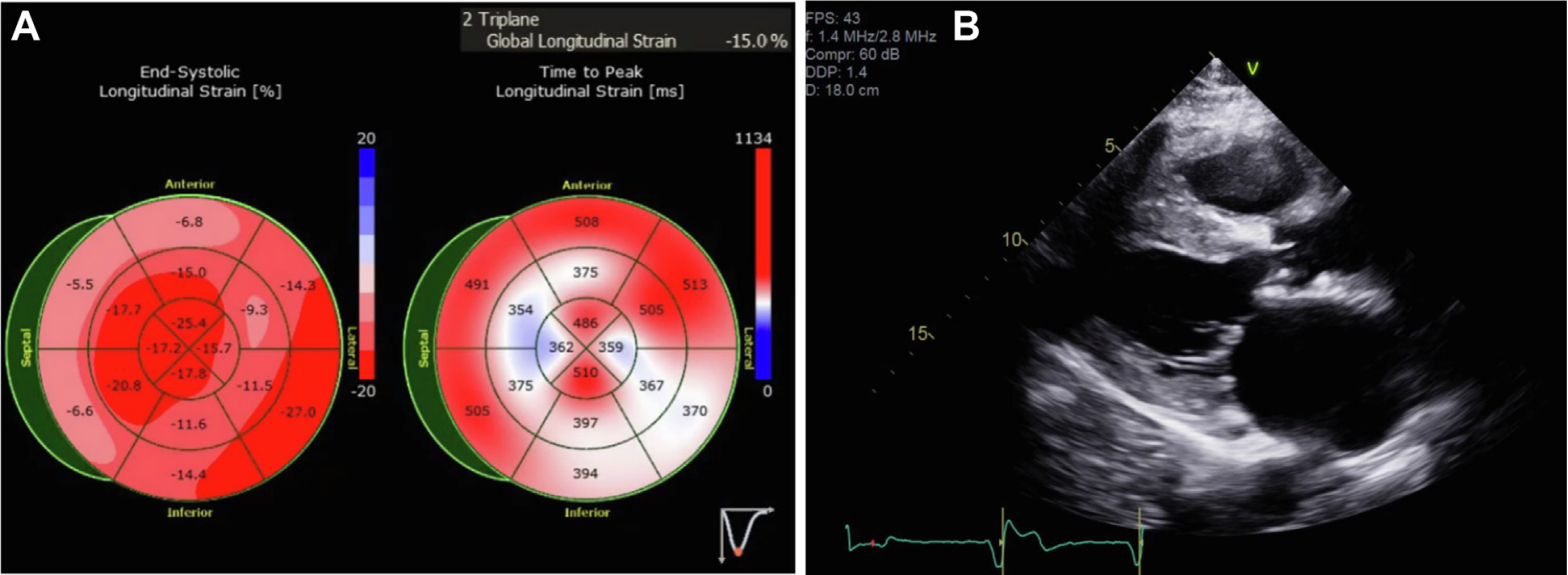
Echocardiogram Images for Patient 1 (A) Patient 1 had reduced global longitudinal strain on the echocardiogram. (B) Transthoracic echocardiogram demonstrating mild symmetrical increase in left ventricular wall thickness (1.3 cm) for patient 1.
